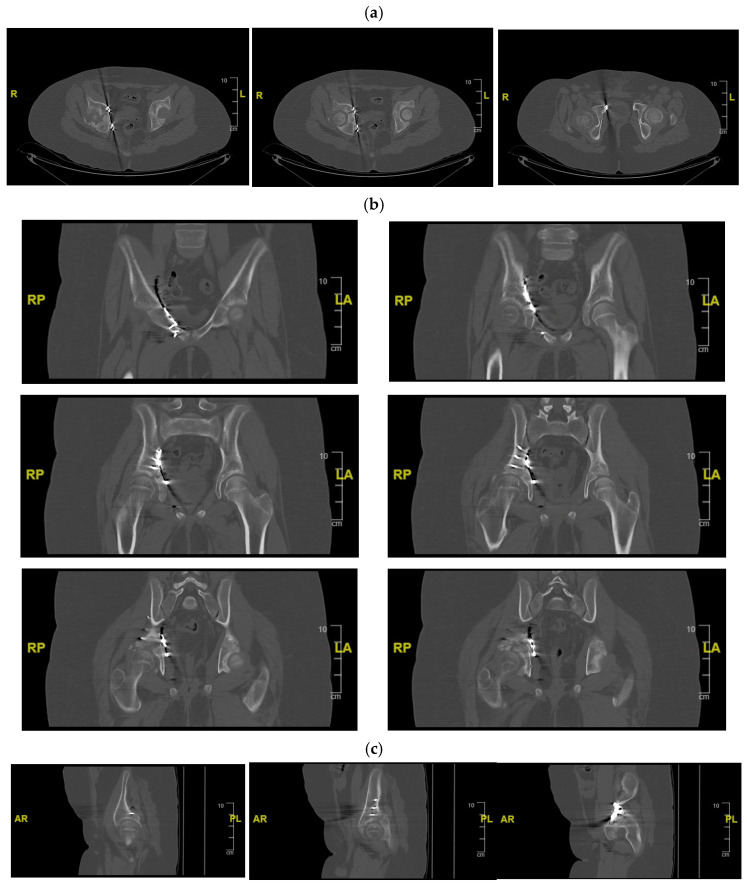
CT of the pelvis showing the synthesis. (a) Axial view; (b) sagittal view; (c) coronal view. R = right; L = left; A = anterior; P = posterior.
